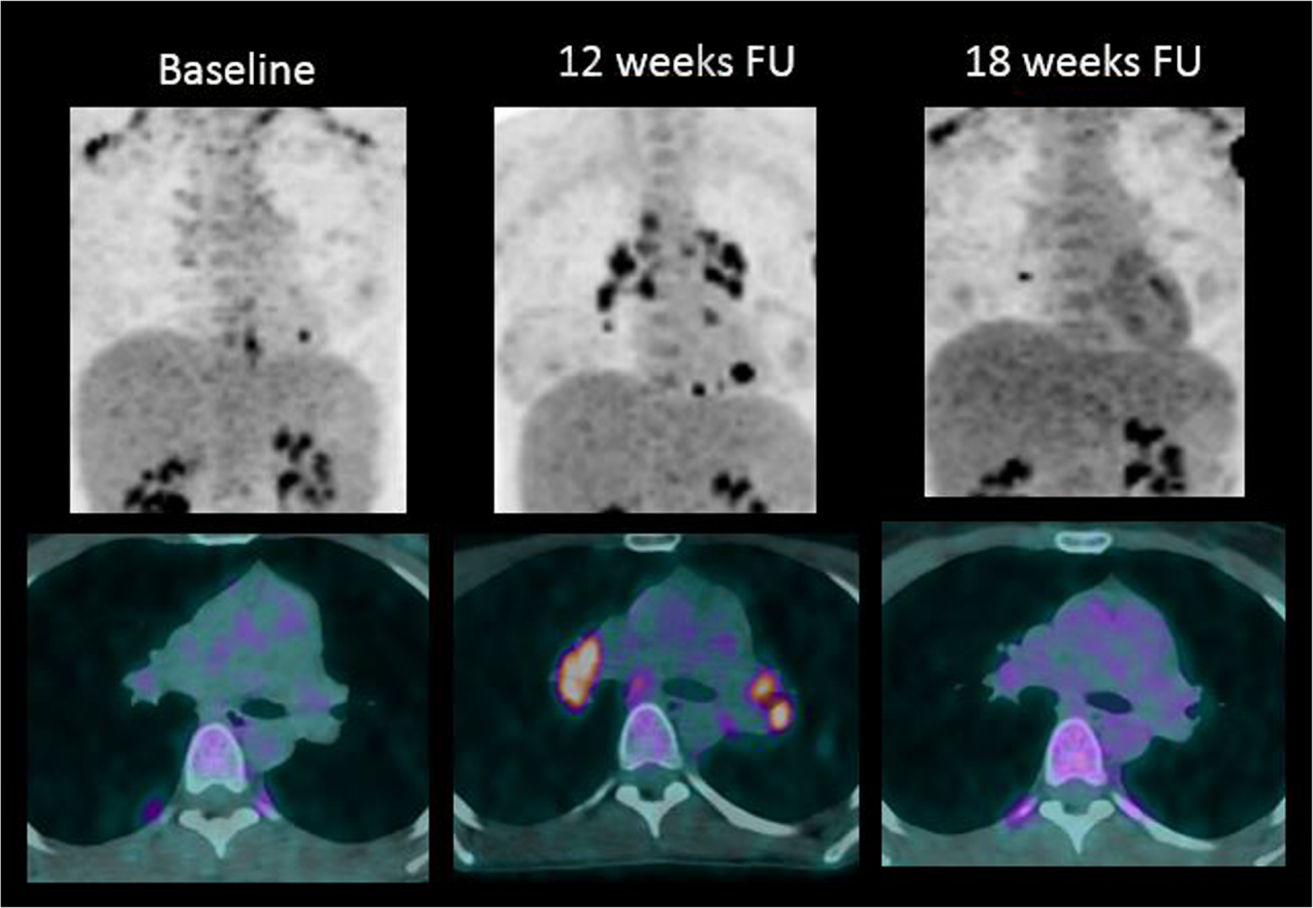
Sarcoid-like reactions in a patient with metastatic melanoma treated with ipilimumab (antiCTLA-4) and nivolumab (anti-PD-1). Twelve-week follow-up (FU) ^18^F-FDG PET/CT images show typical hypermetabolic symmetric mediastinal and hilar lymph node enlargement, very suggestive of a sarcoid-like reaction. These features disappeared on the following ^18^F-FDG-PET/CT images at 18-week FU, confirming the diagnosis of sarcoid-like reaction under immunotherapy
